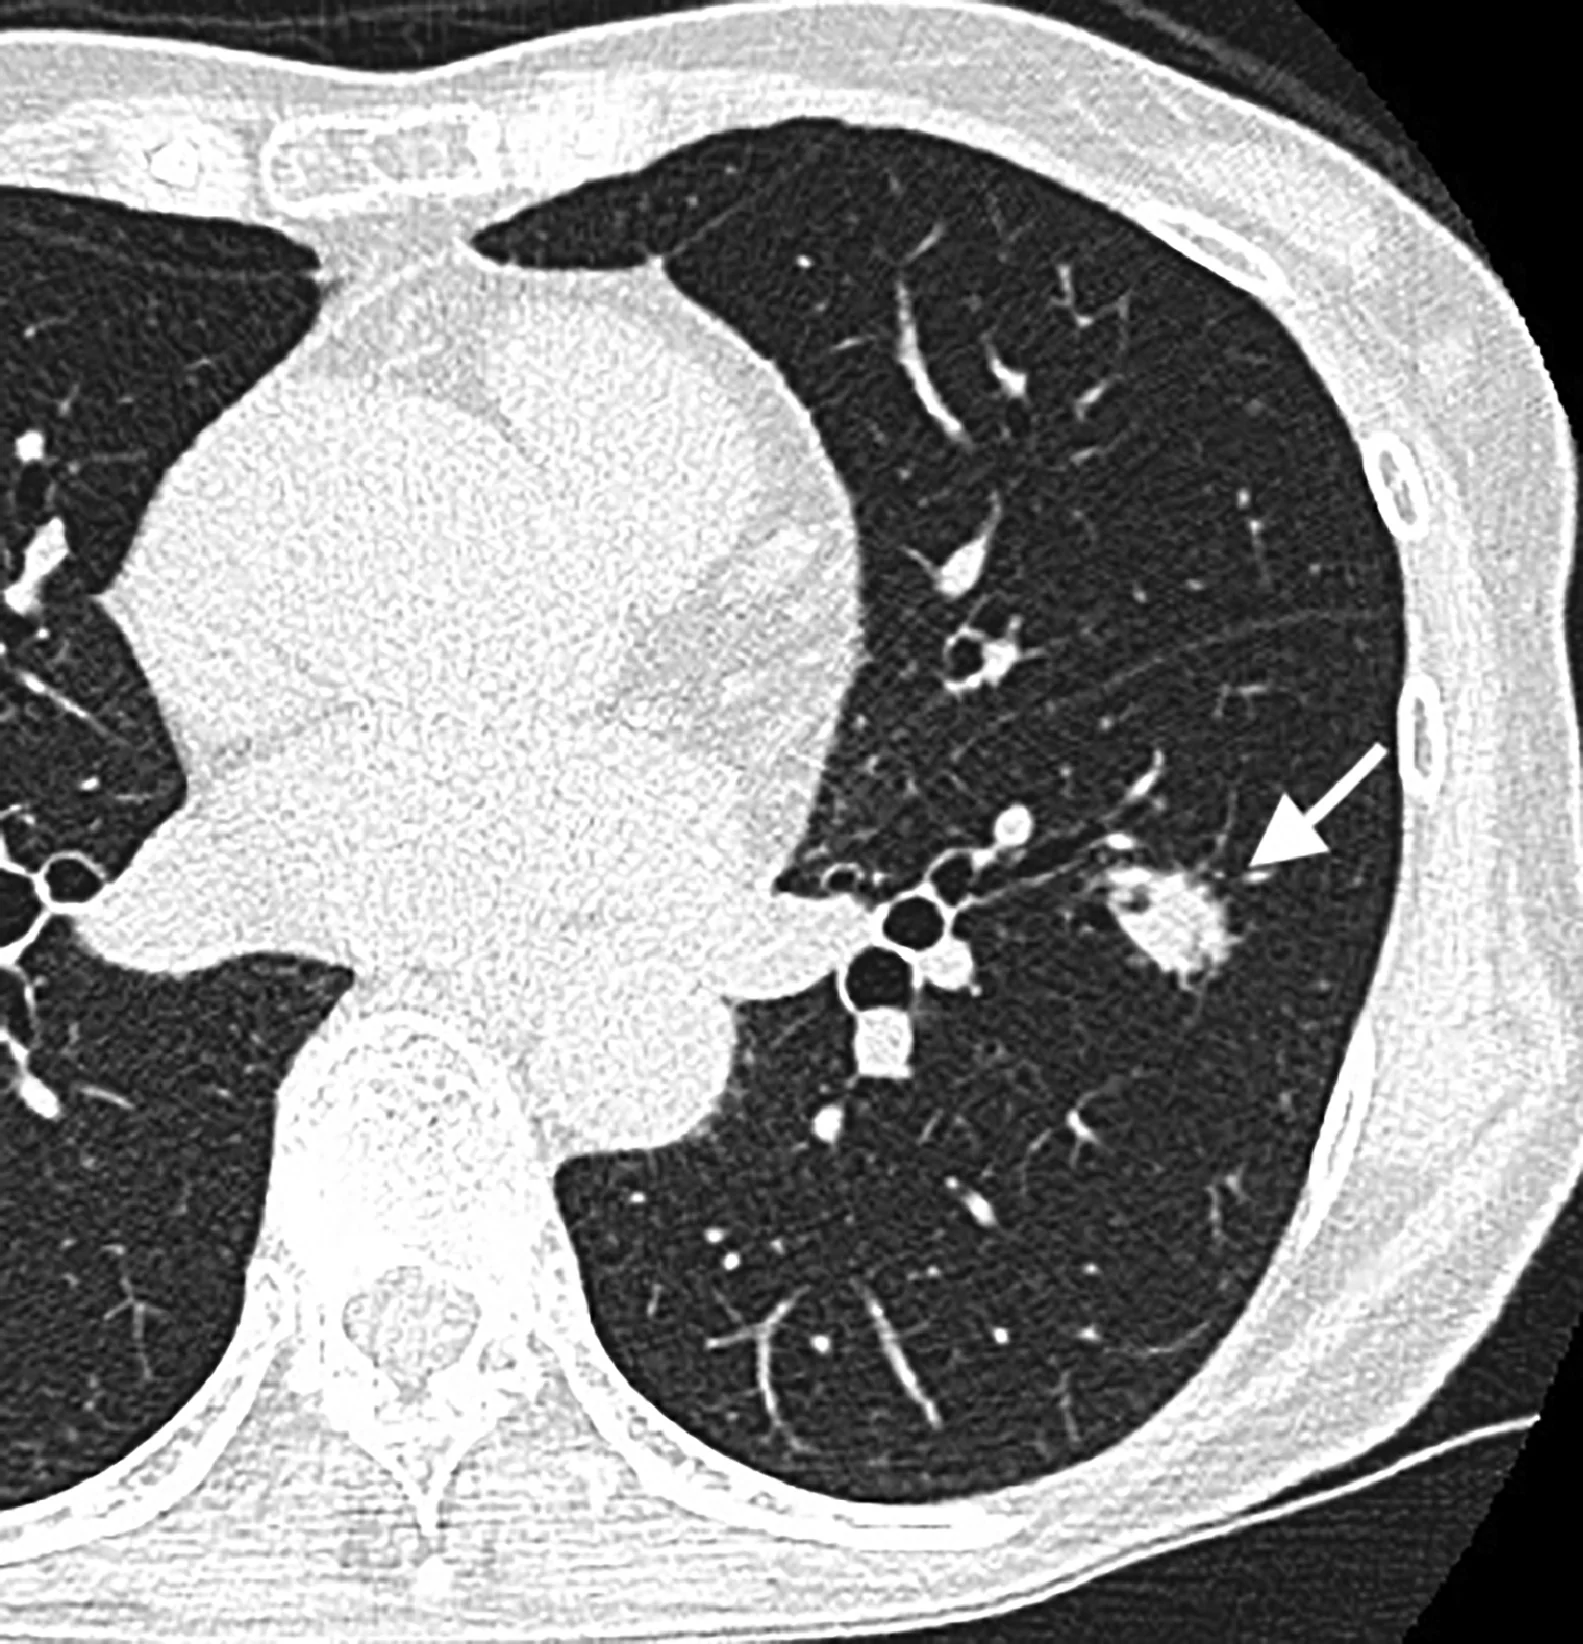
Chest computed tomography (lung window setting) demonstrates a 21 mm × 12 mm solid nodule in segment 10 of the left lower lobe (arrow). Although the margin is relatively well defined, partial spiculation is observed, raising suspicion for primary lung cancer.
